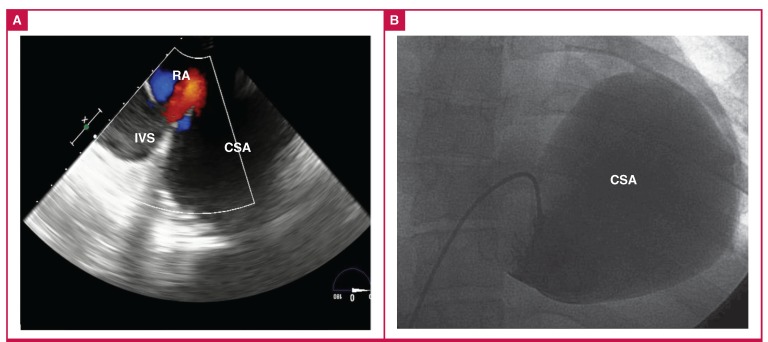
A. Transoesophageal echocardiography colour Doppler flow imaging demonstrated flow from the coronary sinus aneurysm to the right atrium. B. Cardiac catheterisation showed the appearance of the giant coronary sinus aneurysm. RA, right atrium; IVC, inferior vena cava; CSA, coronary sinus aneurysm.
